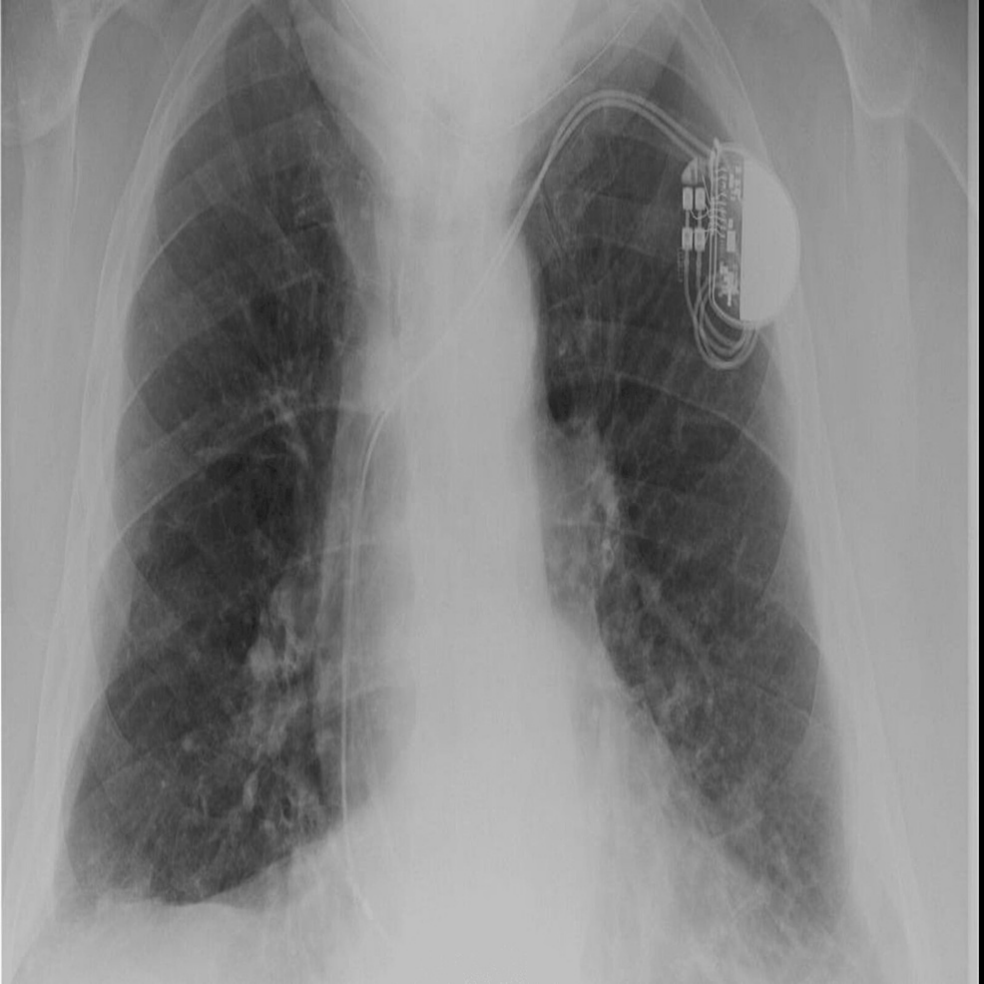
Chest X-Ray showing finding suggestive of left pleural effusion
